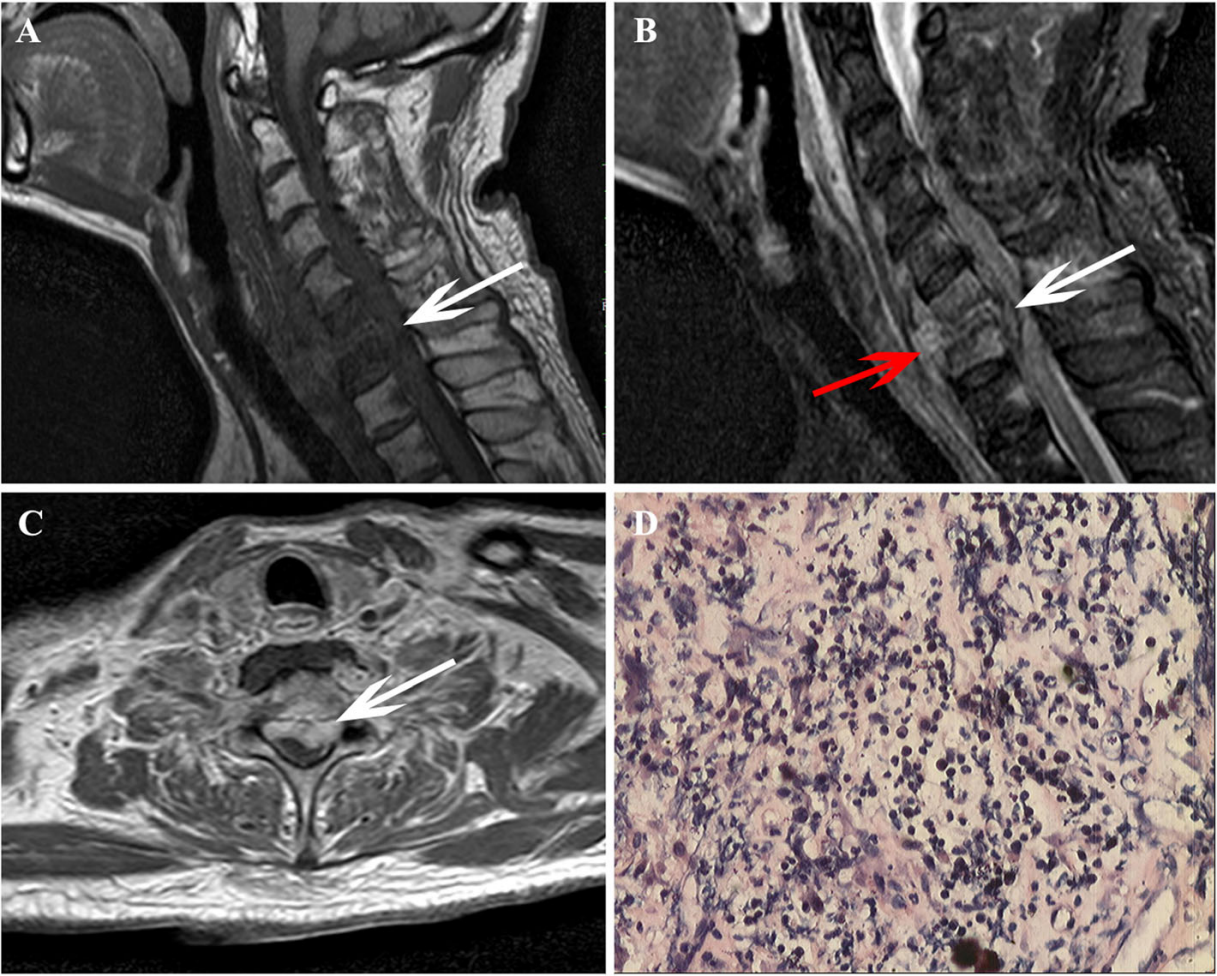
Images in a patient after radiotherapy that was diagnosed with cervical spine ORN by surgery pathology, these images derived from our hospital and patients’ informed consent were waived by the institutional review board. a Sagittal T1-weighted image shows hypointensity change in C6, C7 vertebral body, and paravertebral soft tissue, and the spinal canal is pressed (white arrow). b Sagittal FS T2-weighted image shows hyperintensity change in C6, C7 vertebral body and paravertebral soft tissue, and the spinal canal (white arrow) and prevertebral endorhachis are pressed (red arrow). c Axial CE-T1W image shows marked enhancement of C6 alike soft mass, and the lesion protrudes into the spinal canal (white arrow). d Pathological assessment shows inflammatory cell infiltration was identified in the lesion, without malignancy
